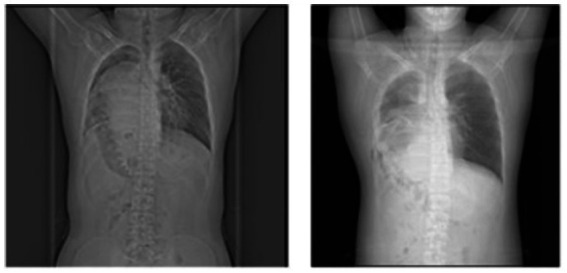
Postoperative chest X-ray showing lung re-expansion.
